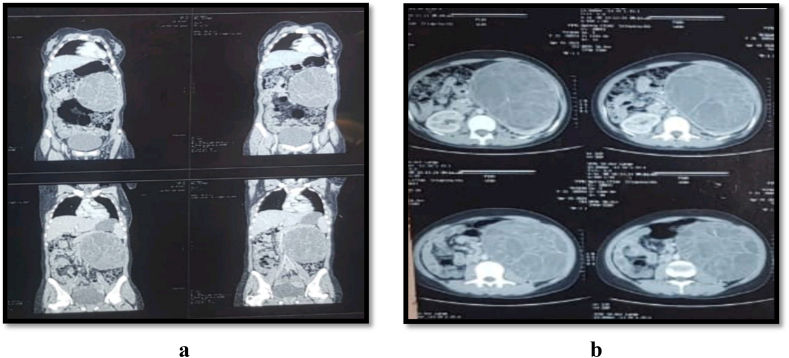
a and b Showing the CT scan imaging of tumor mass involving left renal tissue in different planes.
